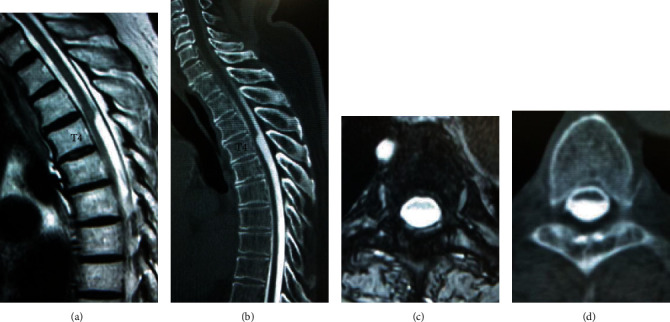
Sagittal MRI and CT myelography demonstrate a dorsal indentation, the so-called “scalpel sign,” at the T4 level.
